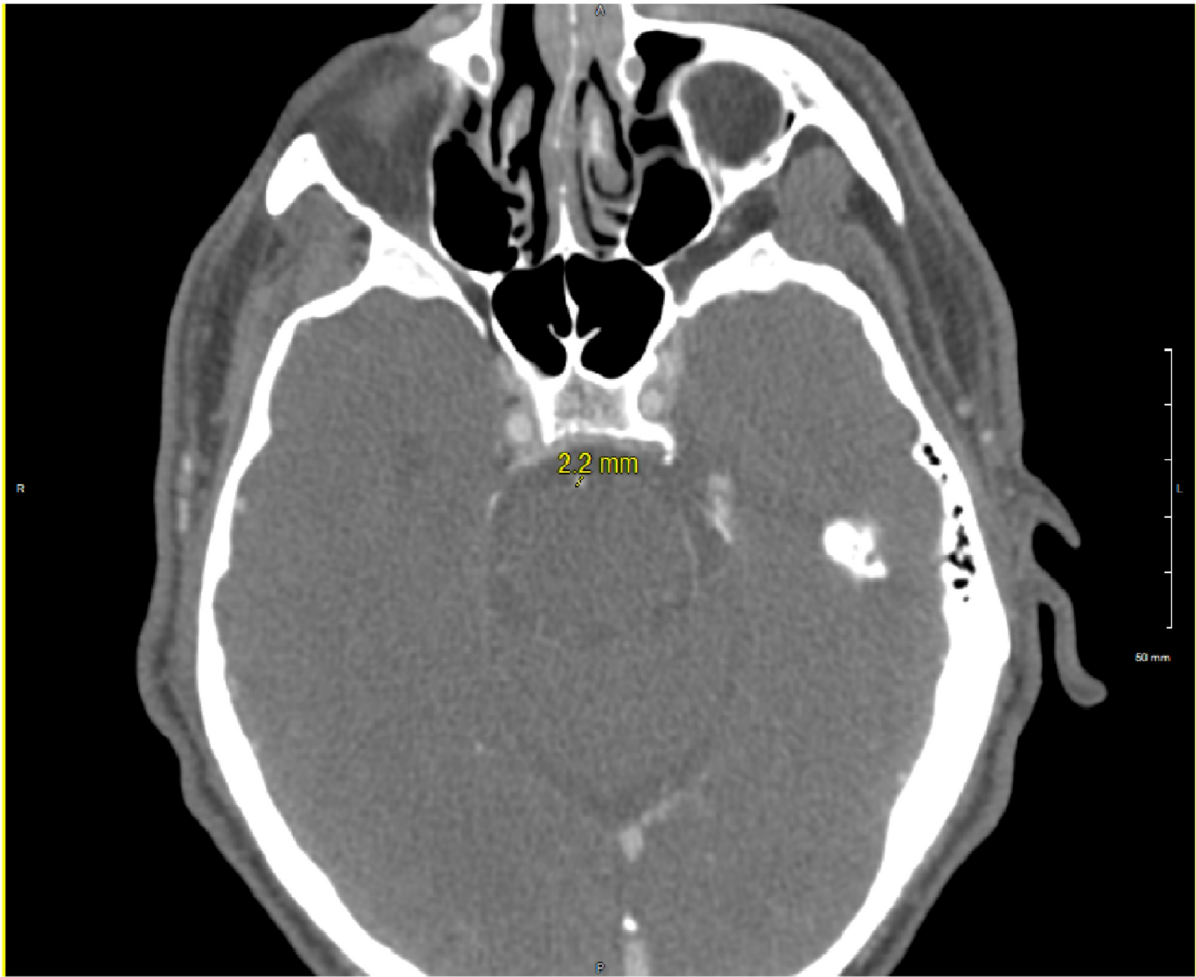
CT angiography (CTA) head performed on hospital day 1 showing a small caliber basilar artery measuring at 2.2 mm.
